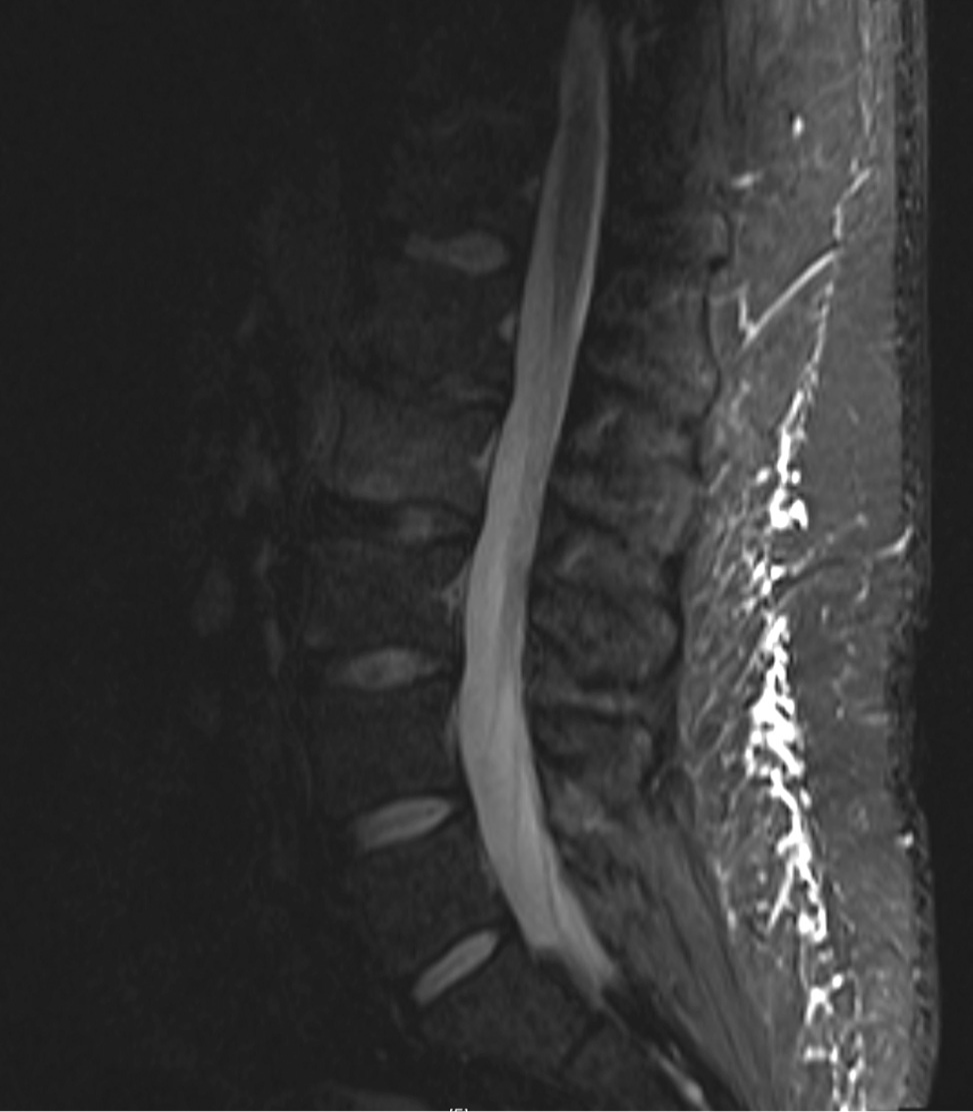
Initial MRI with contrast showing increased STIR signal change indicating diffuse bone marrow edema. STIR - short T1 inversion recovery
